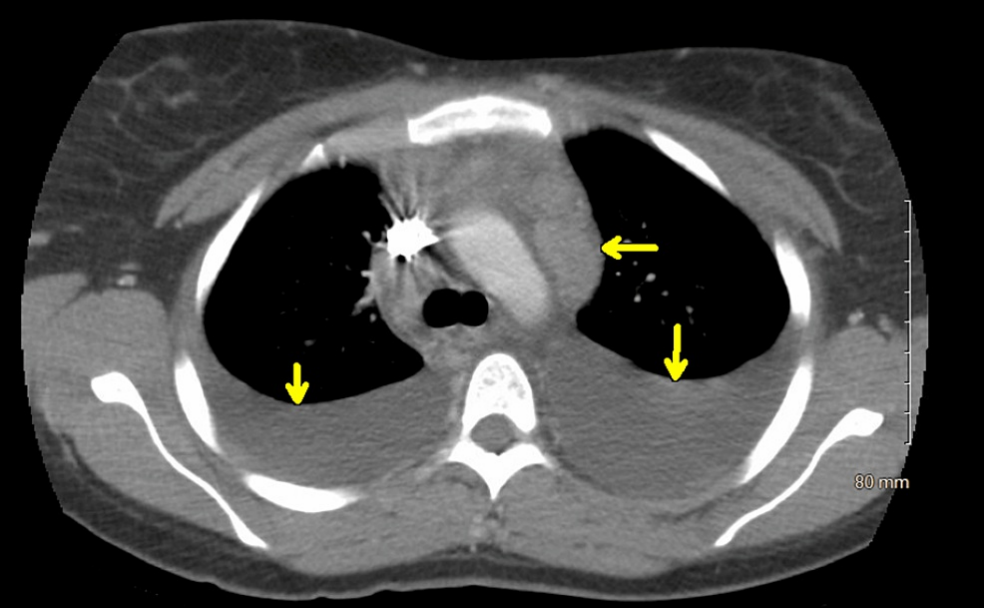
Computed tomography scan image of the chest with bulky intrathoracic adenopathy Arrows indicate bulky intrathoracic adenopathy.
